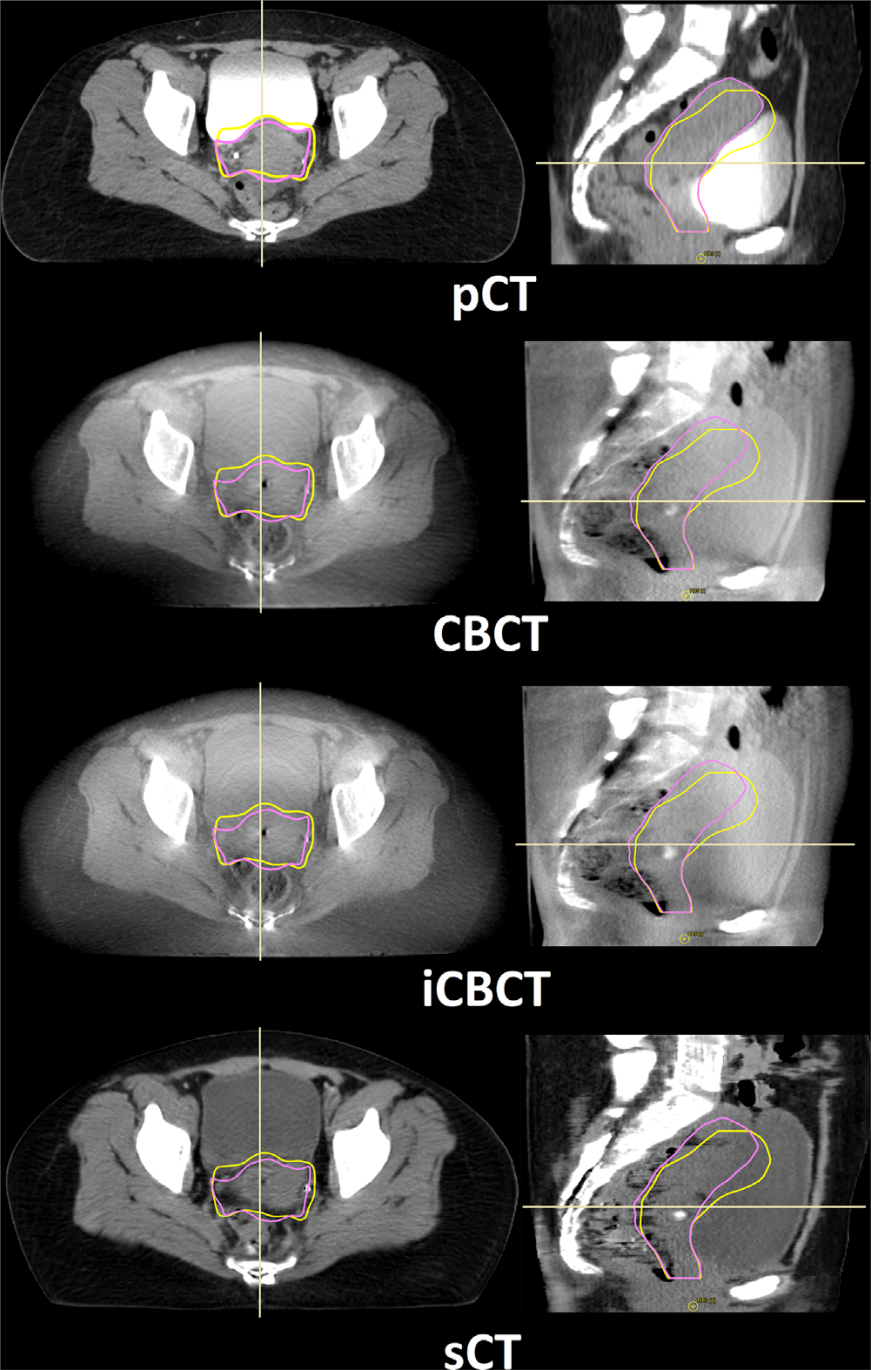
A typical example of the different types of images from one patient. From top to bottom, planning CT, XVI CBCT, iterative reconstructed CBCT generated with FRESCO and synthetic CT image generated from XVI CBCT with ADMIRE. Left to right in each row, transversal view and sagittal view. The yellow line is the CTV for the empty bladder plan and the pink line is the CTV for the full bladder plan.
